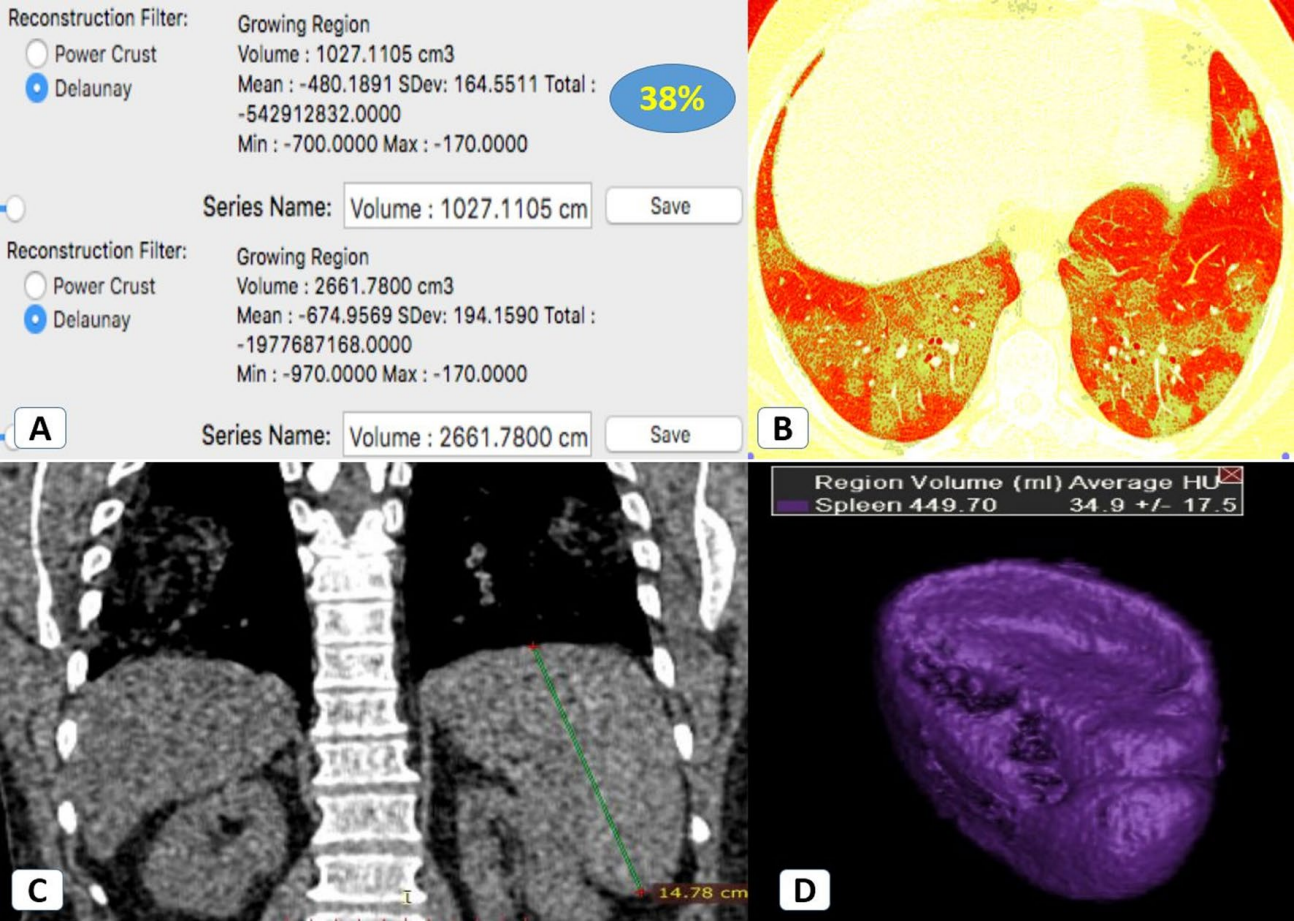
Splenomegaly in a 58-year-old female patient with severe COVID-19 infection: A, B Volumetric CT assessment of the lung revealed 38% pathological lung involvement. C Coronal CT mediastinal window showed an increased craniocaudal bi-polar diameter of the spleen (14.8 cm). D CT volumetry of the spleen was 450 cc
